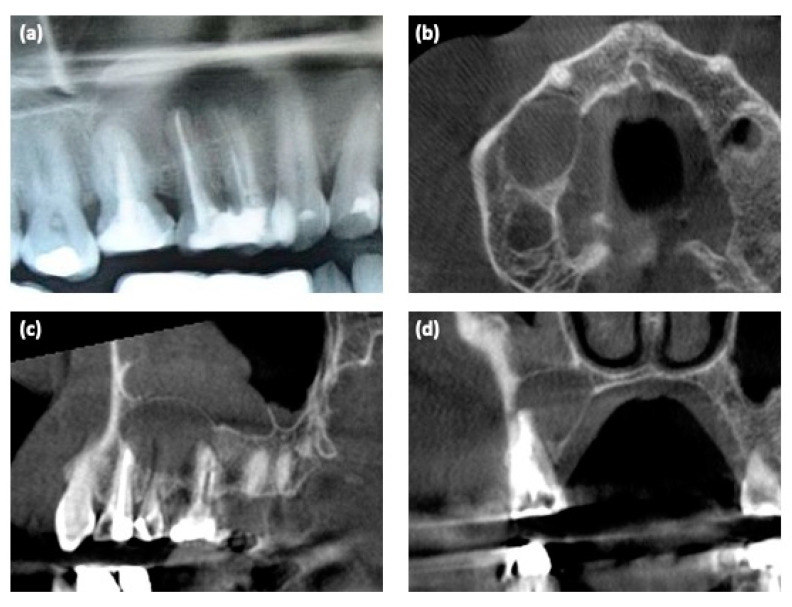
Radiological examinations. (a) Detail of the dental X-ray at the first clinical examination; (b–d) 3D CBCT scan before surgery.
